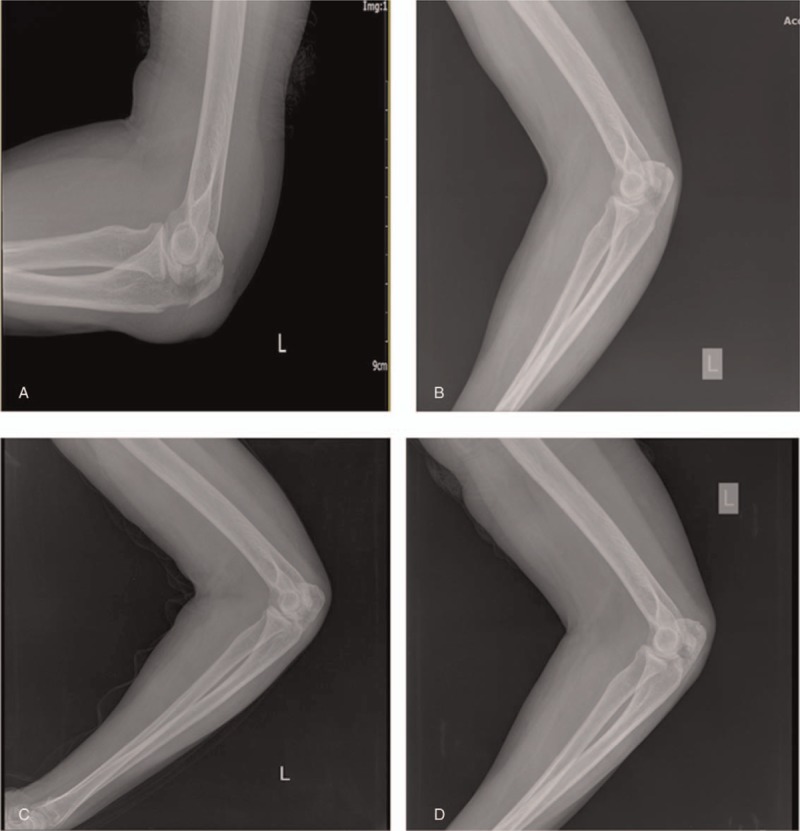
X-ray images showing a displaced fracture of the left olecranon with soft tissue swelling at the initial visit on December 11, 2016 (A) and at the follow-up visits after initiation of treatment on January 5, 2017 (B), February 2, 2017 (C), and March 16, 2017 (D).
